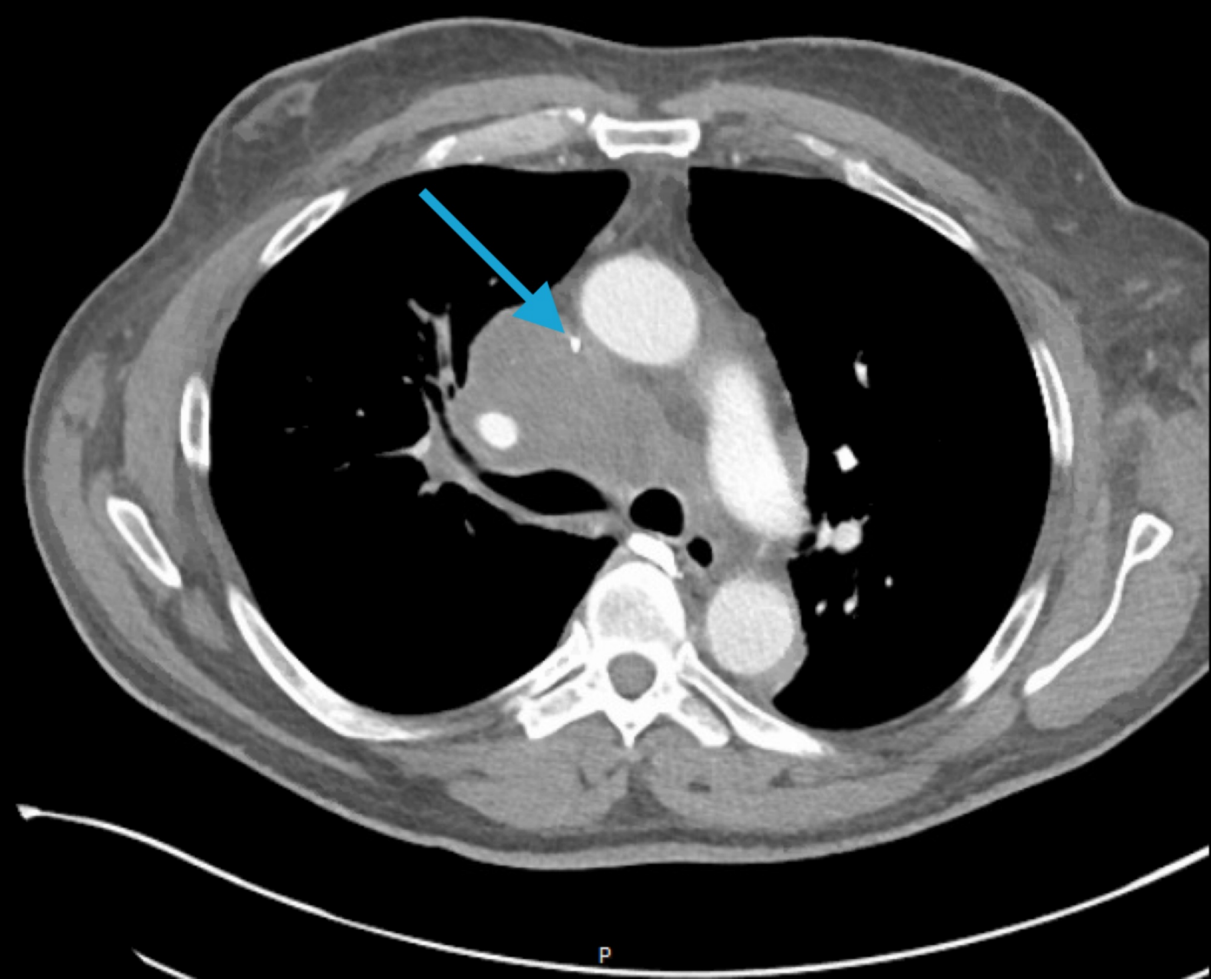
Computed tomography (CT) scan of the chest showing the right paratracheal mass encasing the superior vena cava (SVC) (indicated by blue arrow).
